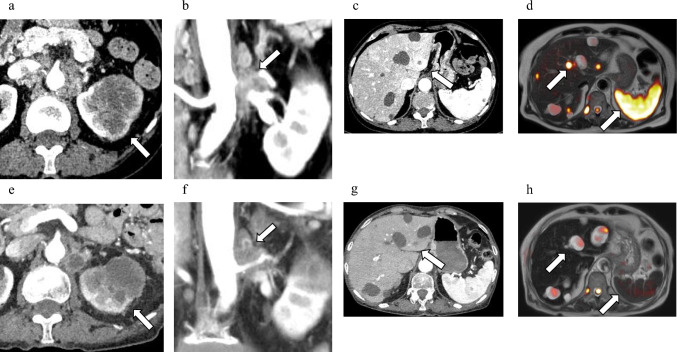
Imaging examinations of Case 2 before and after combined nivolumab and cabozantinib treatment. a Compute tomography (CT) showing primary left renal tumor before combined treatment. b CT showing para-aortic lymph node metastatic lesions before combined treatment. c CT showing liver metastatic lesion before combined treatment. d Diffusion-weighted imaging with background suppression (DWIBS) showing liver and splenic metastatic lesions before combined treatment. e CT showing the primary left renal tumor after combined treatment. f CT showing para-aortic lymph node metastatic lesions after combined treatment. g CT showing liver metastatic lesions after combined treatment. h DWIBS showing liver and splenic metastatic lesions after combined treatment
